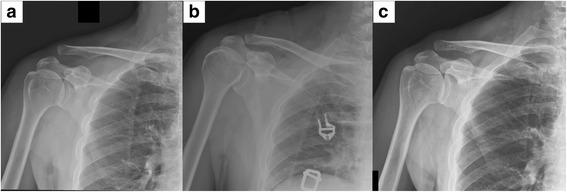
Reduction loss after coracoclavicular fixation with Mersilene tape. a Preoperative plain radiograph. b 1-month postoperative follow-up. c 6-month postoperative follow-up with reduction failure
